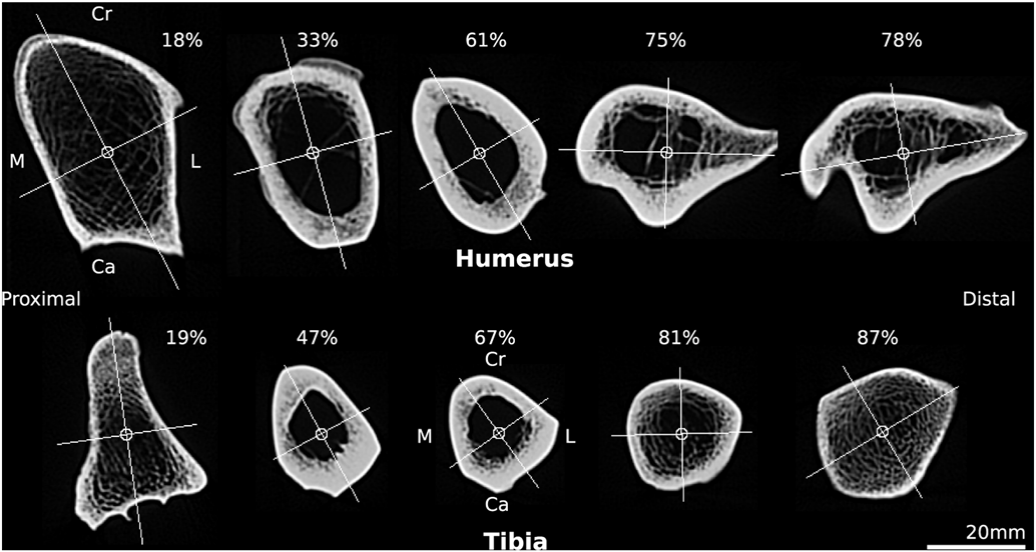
Computed tomograms of a tiger humerus and tibia. Selected CT slices from the humerus and tibia of *Panthera tigris* (tiger) with centroids and principal axes shown. Note the substantial variation in cortical shape and thickness along the length of each bone. Per cent length is indicated for each slice; 0% is most proximal. Cr, cranial; Ca, caudal; M, medial; L, lateral.
